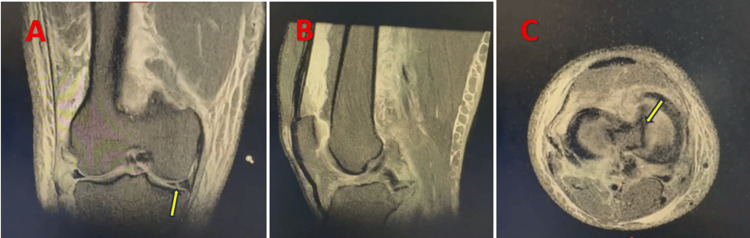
Radiological findings (MRI) confirming the meniscal lesion. (A) Coronal slice through the meniscus showing the lesion (yellow arrow)
